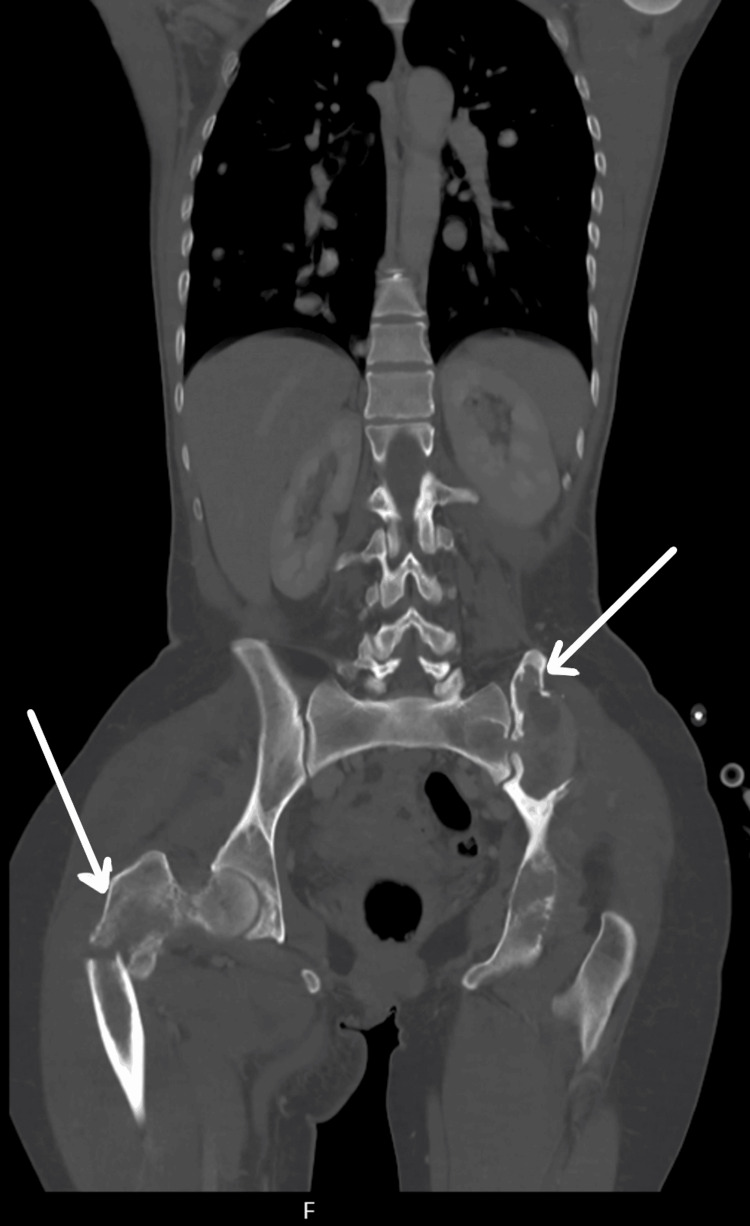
Computed tomography of the thorax, abdomen, and pelvis showing a lytic lesion in the right proximal femur alongside a left pelvic lesion
